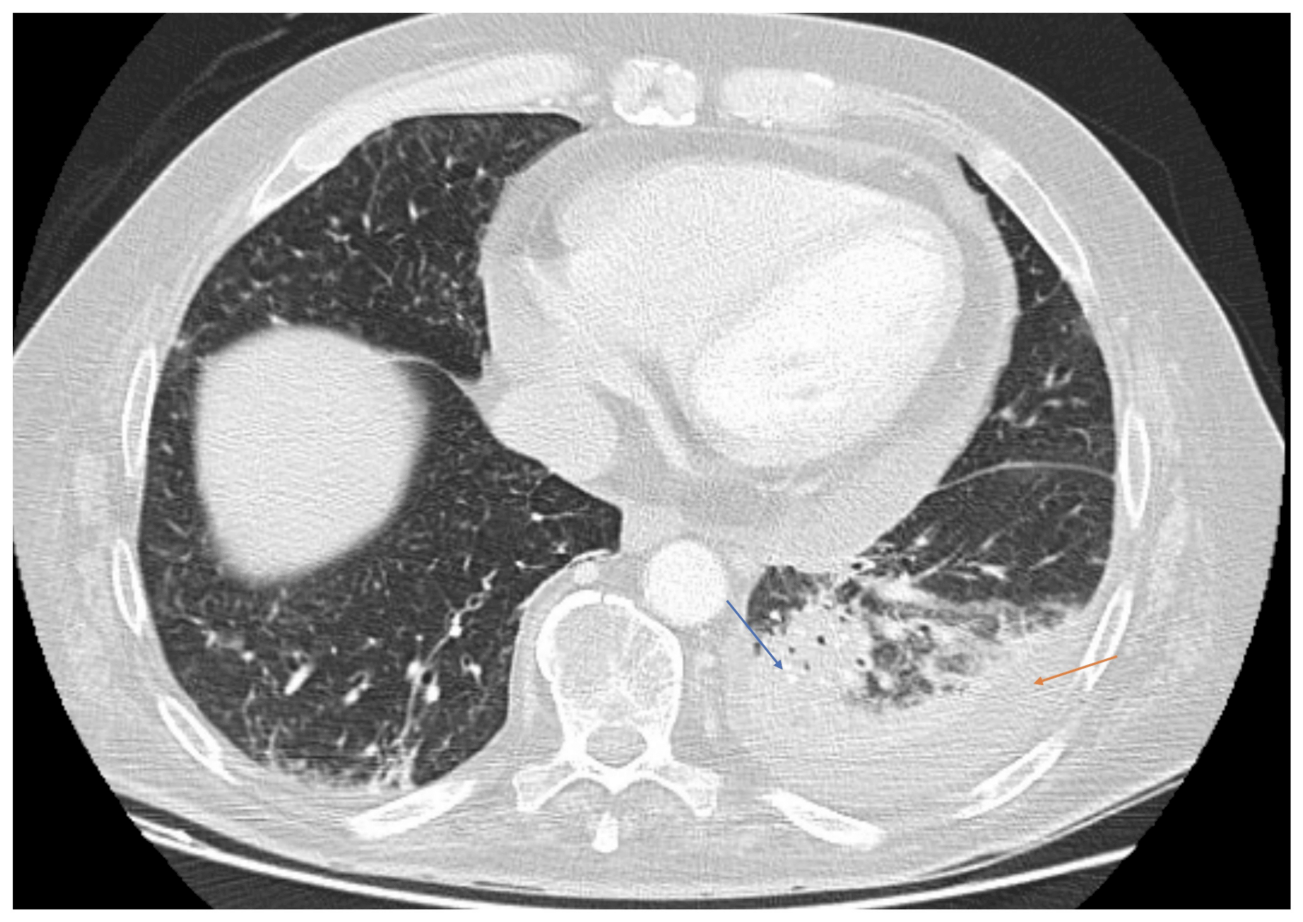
Contrast-enhanced CT scan of the chest showing alveolar infiltrates in the lower lobe of the left lung (blue arrow) with adjacent pleural effusion (orange arrow)
